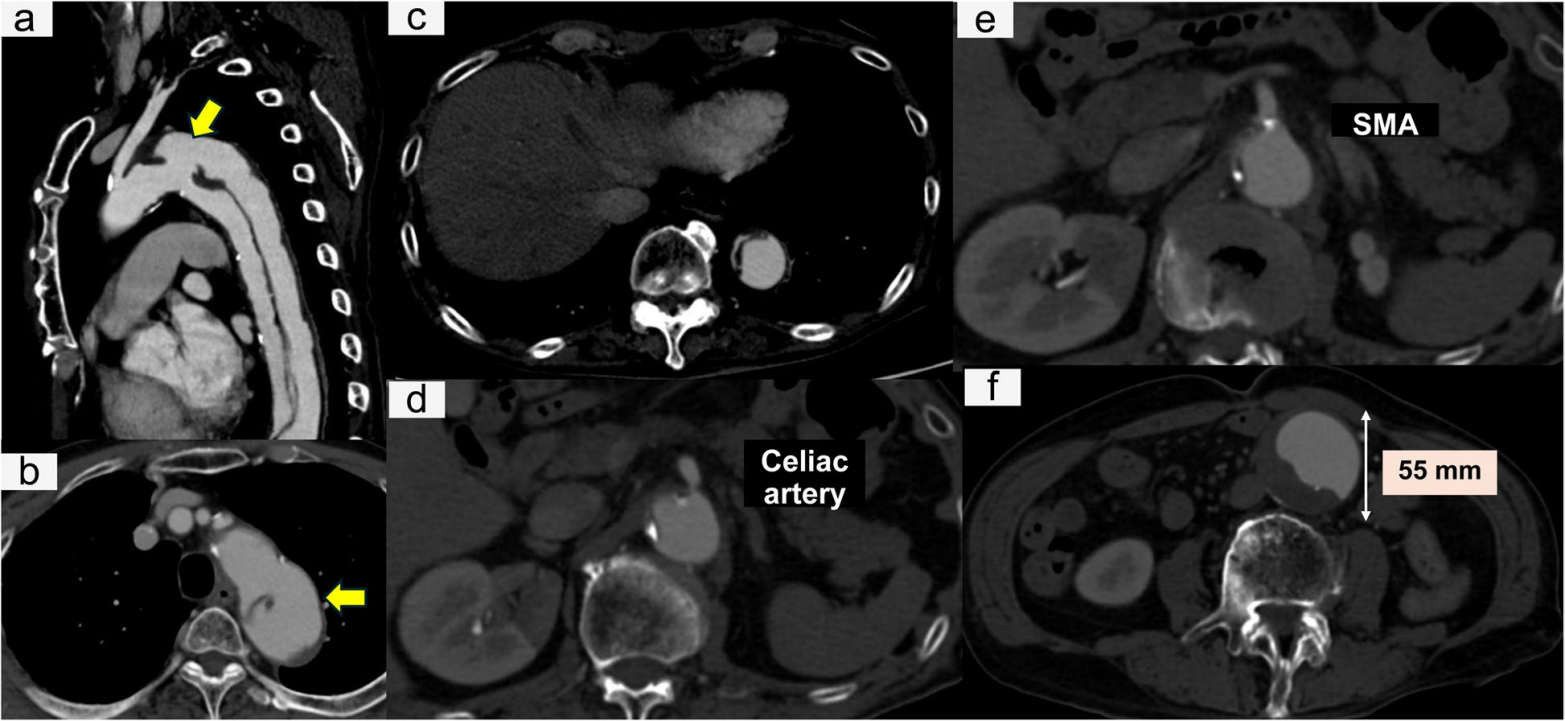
Preoperative computed tomography images. Sagittal (a) and axial (b) images show the entry tear (yellow arrow) in the descending aorta. Axial images reveal a severely compressed TL (c, d, e), the celiac artery originating from the FL (d), the SMA arising from the narrowed TL (e), and a 55-mm abdominal aortic aneurysm (f). TL, true lumen; FL, false lumen; SMA, superior mesenteric artery
